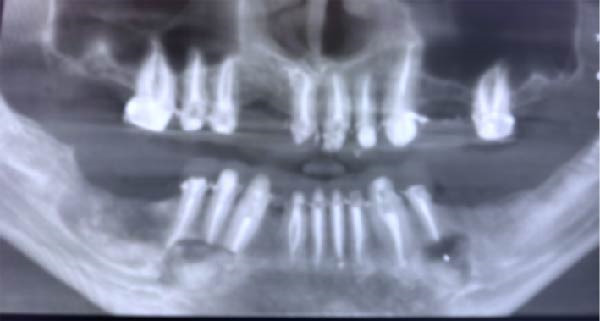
Panoramic radiograph after lesions enucleation, placement of the collagen membrane and retreatment of the whole lower teeth.
